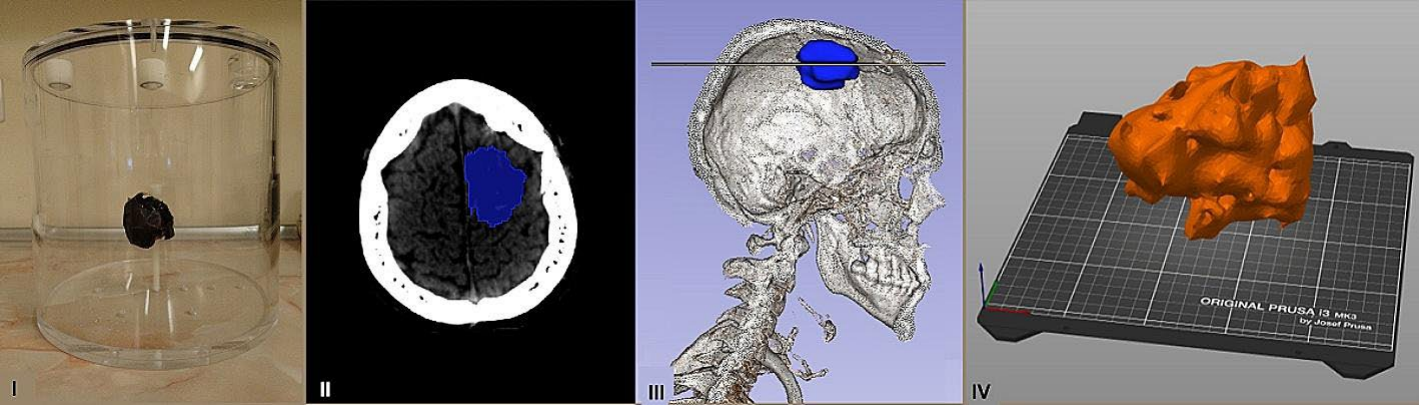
The 3D printed model and its positions in the main cylinder (I and IV). The 3D printed model was created based on CT scan: clinical target volume (II). 3D printed model located in the patient’s brain (III). The line in (III) represents the CT scan shown in (II)
